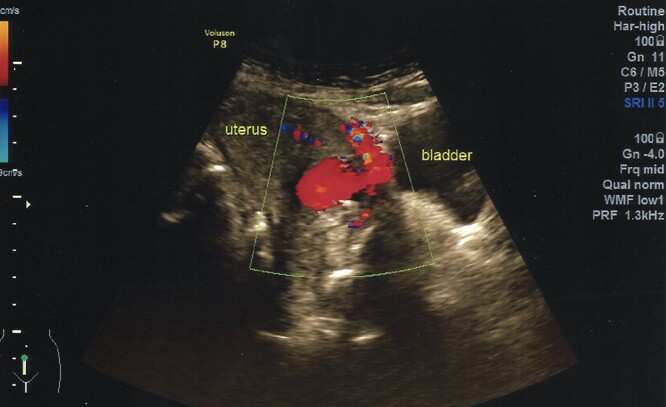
Ultrasonography demonstrates a rich blood flow in the lower uterine segment with a normal fundus.
